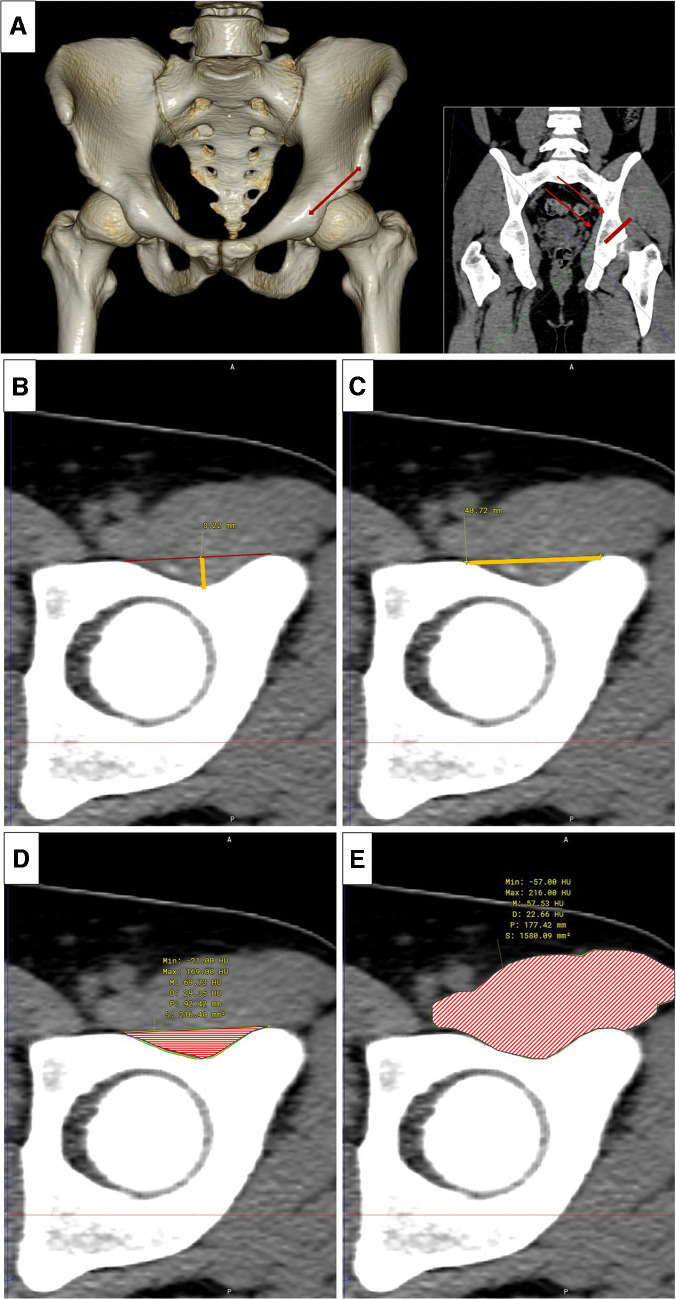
Identification of the borders of the psoas valley on 3D reconstructed CT scans (A). After aligning on the identified border, the valley’s depth (B) and width (C) were measured on the axial plane (orange lines). The cross-sectional area of the valley (D) and the muscle on top (E) were then measured on the same plane (red dashed area)
